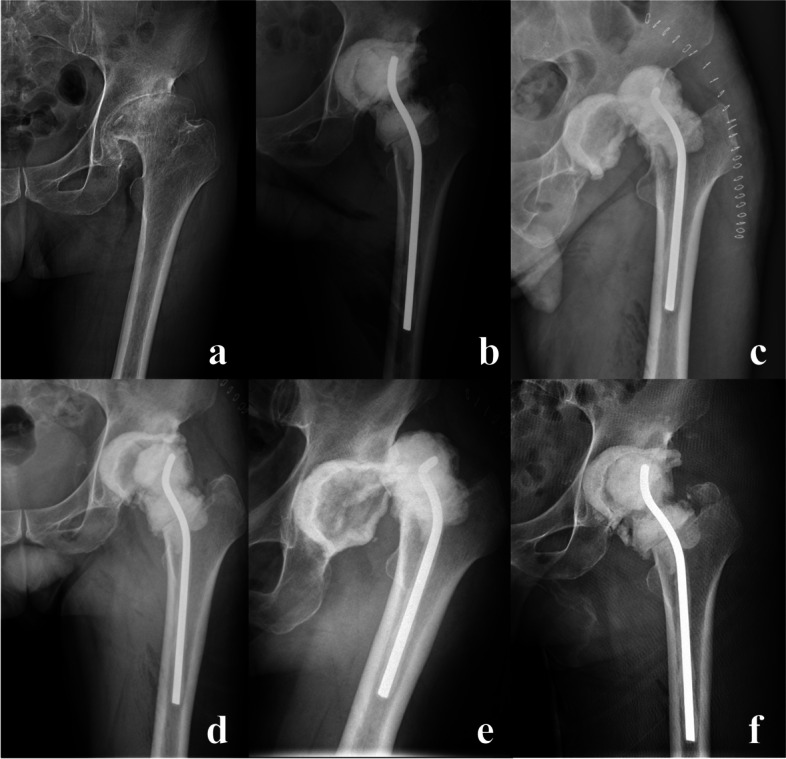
One case of periprosthetic fracture. A 67-year-old male patient was diagnosed with a purulent infection of the left hip and underwent debridement and antibiotic-loaded bone cement temporary spacers. PD occurred twice, and closed reduction was successful with the Allis maneuver. However, periprosthetic fracture of the greater trochanter was confirmed after the second reduction and treated with a conservative strategy because the spacers were stable. a. Preoperative X-ray film of the affected hip. b. Anteroposterior (AP) X-ray film of the affected hip immediately after revision. c. AP view X-ray film of the affected hip immediately after the first PD. d. AP view X-ray film immediately after the first reduction. e. AP view X-ray film immediately after the second PD. f. AP view X-ray film after the second reduction, showing greater trochanter fracture
